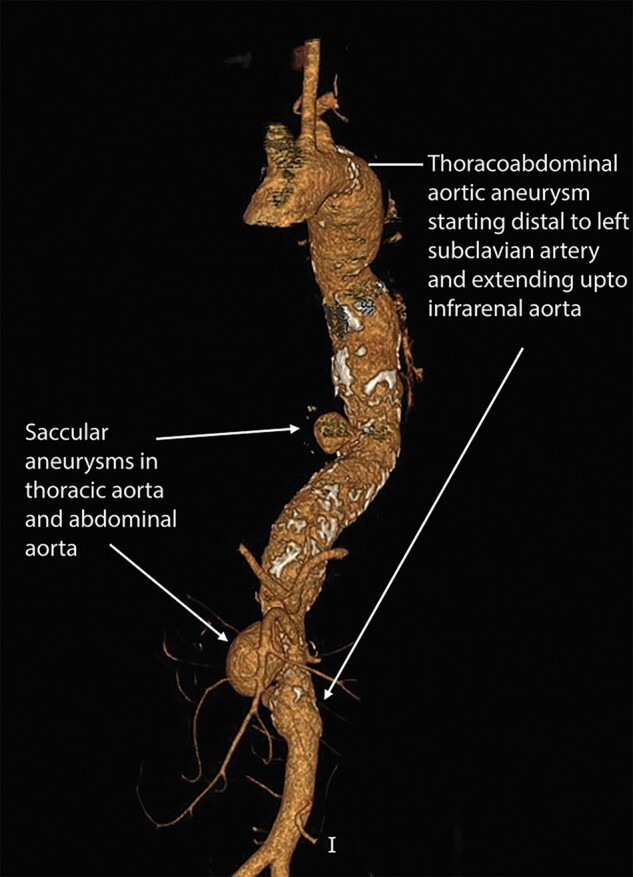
CT aortography. 3D reconstructed image showed extensive (type II) thoracoabdominal aortic aneurysm extending from the left subclavian artery to the infrarenal aorta. Aortic arch branches were normal.
